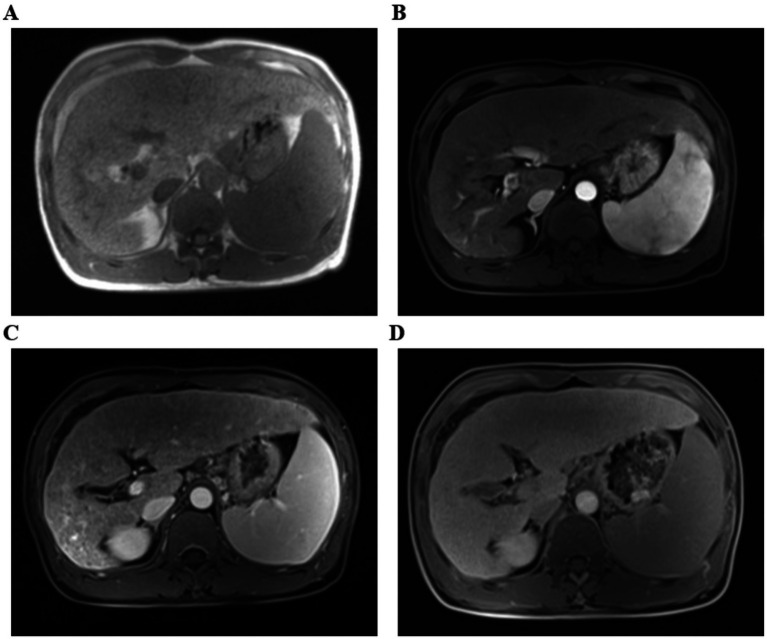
The upper abdominal magnetic resonance imaging (MRI) with T1-weighted sequences. (A) Flat phase. (B) Arterial phase. (C) Portal venous phase. (D) Delayed phase. (The arrow indicates that it suggest liver cirrhosis).
